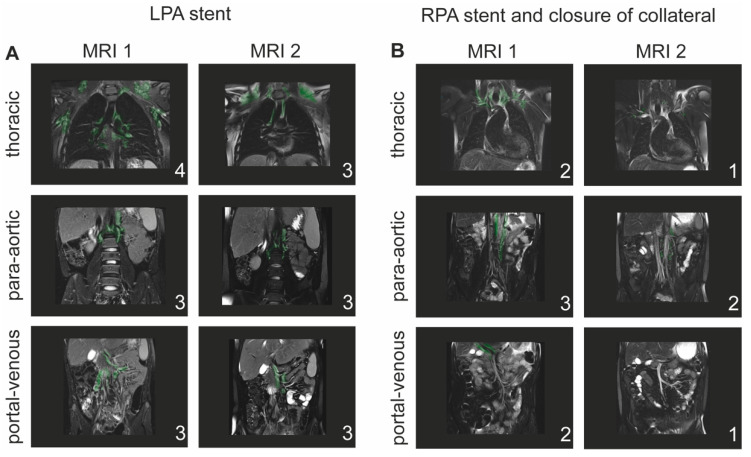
Example of lymphatic abnormalities after cardiac catheter intervention. (A) Patient who underwent first cardiac MRI at 13 years of age showing moderate left pulmonary artery (LPA) stenosis. The T2-weighted imaging showed significant thoracic lymphatic abnormalities (type 4) and para-aortic and portal-venous abnormalities type 3. The 2nd MRI was performed after cardiac catheterization and LPA stent implantation showing mildly improved thoracic lymphatic abnormalities. (B) 25-year-old Fontan patient in good clinical condition presented with significant stenosis of the right pulmonary artery (RPA) on 1st MRI. Cardiac catheter intervention with balloon dilatation of the RPA and closure of a major aortopulmonary collateral (MAPCA) from the descending aorta was performed. Repeat MRI at the age of 30 years showed improvement in lymphatic abnormalities in all three compartments. Lymphatic abnormalities are highlighted in green.
